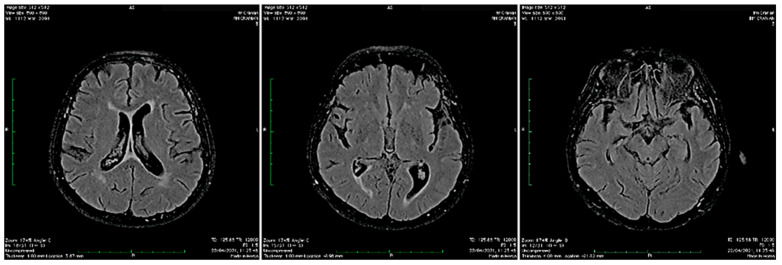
Cerebral MRI, FLAIR sequences revealing mild periventricular microvascular changes and cerebral atrophy.
